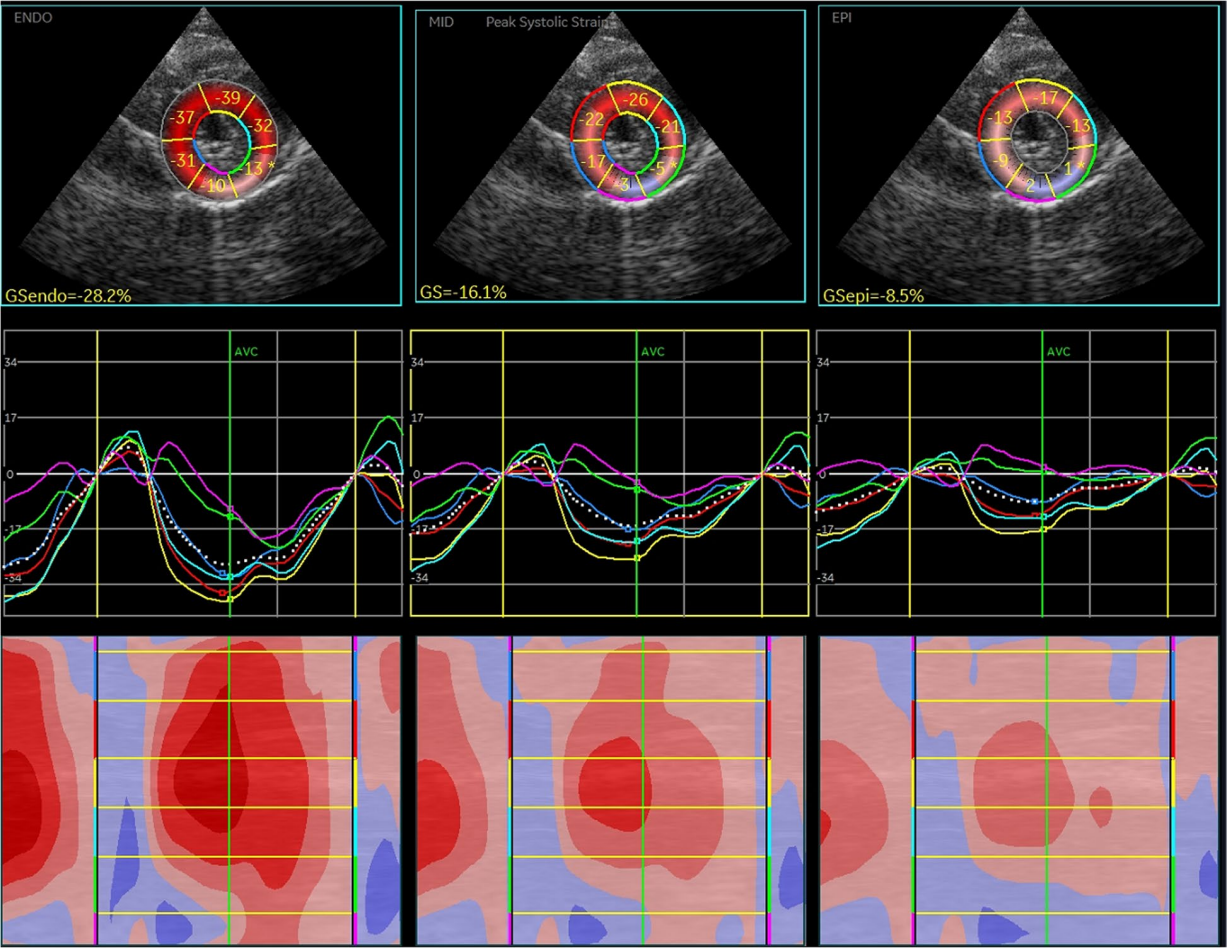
Two-dimensional speckle-tracking echocardiography (on the 4th day after diagnosis). The green lines indicate myocardial deformations of the left ventricular free wall. This site corresponds to the site of myocardial hypertrophy and cardiac needle biopsy. From left to right: results for the endomyocardial layer, whole layer of the myocardium, and the epimyocardial layer. Note the significant decrease in green (segmental strain value is -5%) and pink myocardial site (segmental strain value -3%) in whole layer strain (center)
